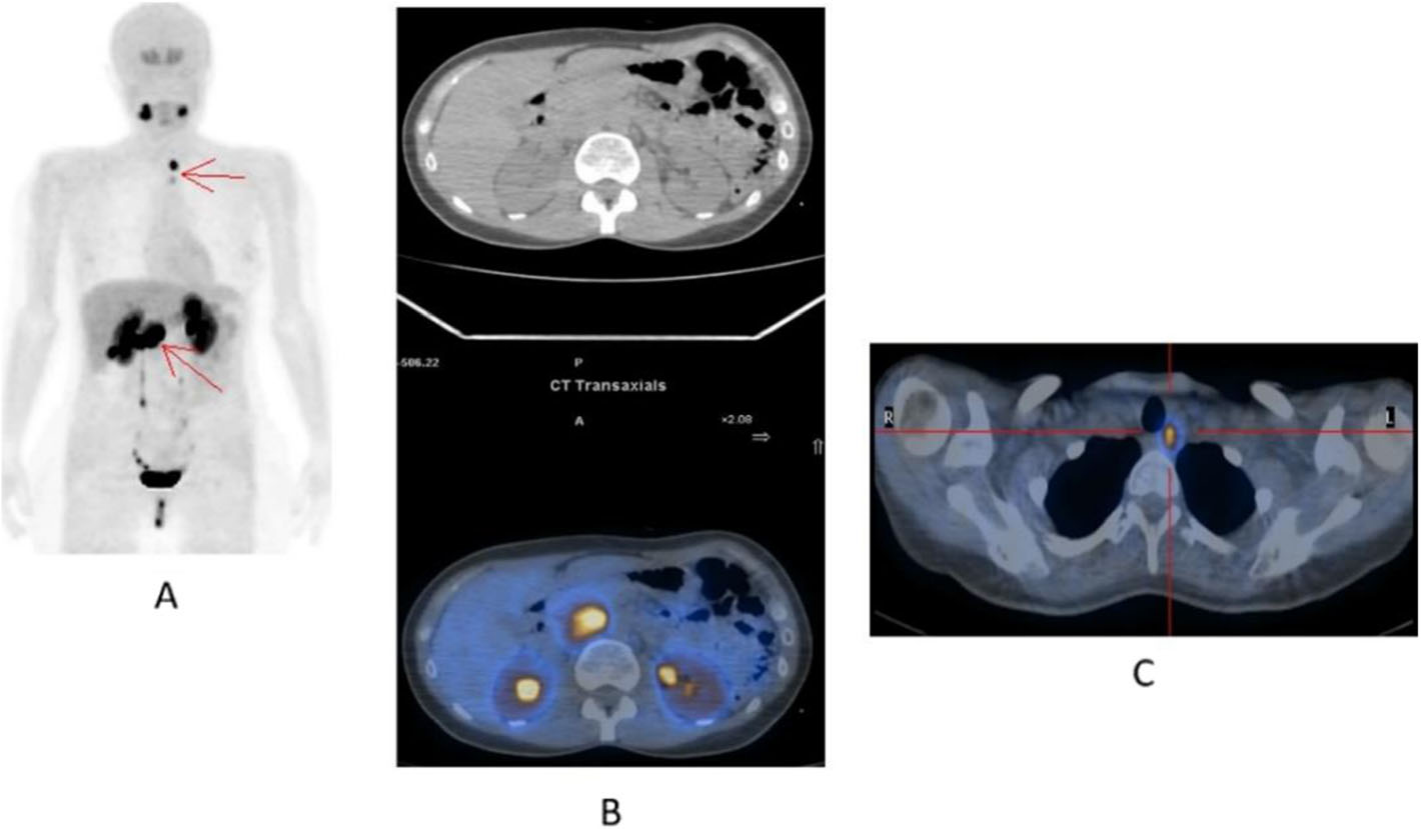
^18^F-FDOPA, paraganglioma. *Clinical history*: 48 y.o. man with suspected abdominal paraganglioma. *PET/CT findings*: intense uptake of the tracer in the abdominal paraganglioma and in a left paratracheal lesion showed in MIP (a), CT and fused images (b and c). Surgery confirmed two paraganglioma lesions
